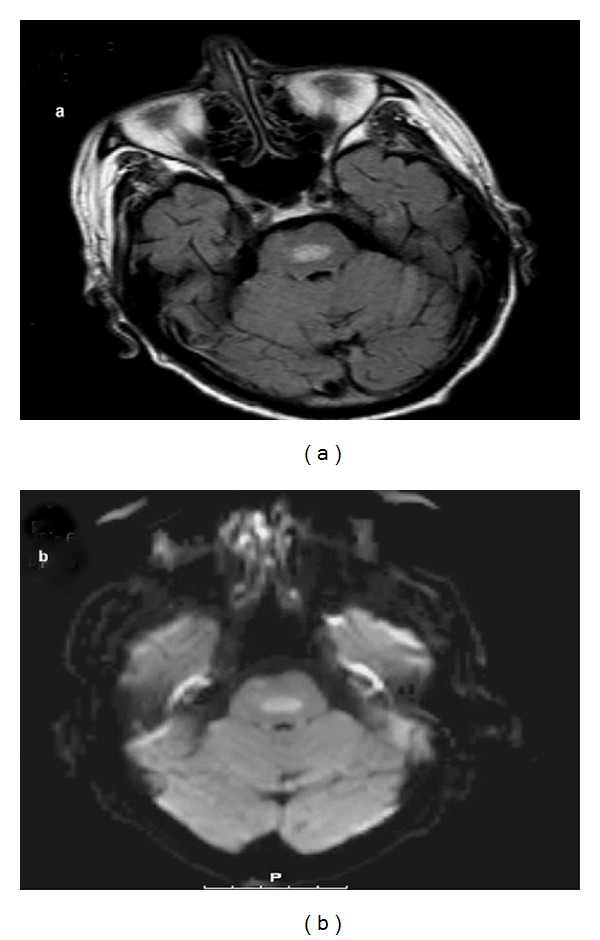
Ill-defined FLAIR hyperintense lesion seen in central pons (a) and restricted diffusion noticed in pons on DWI sequence (b).
